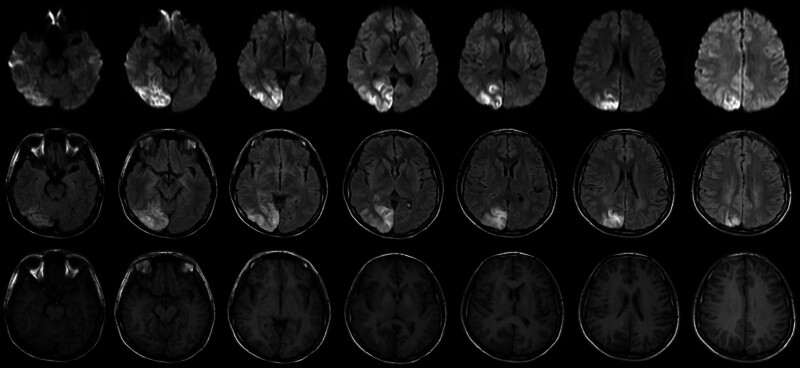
The patient’s brother underwent a cranial MRI on January 14, 2024. MRI = magnetic resonance imaging.
